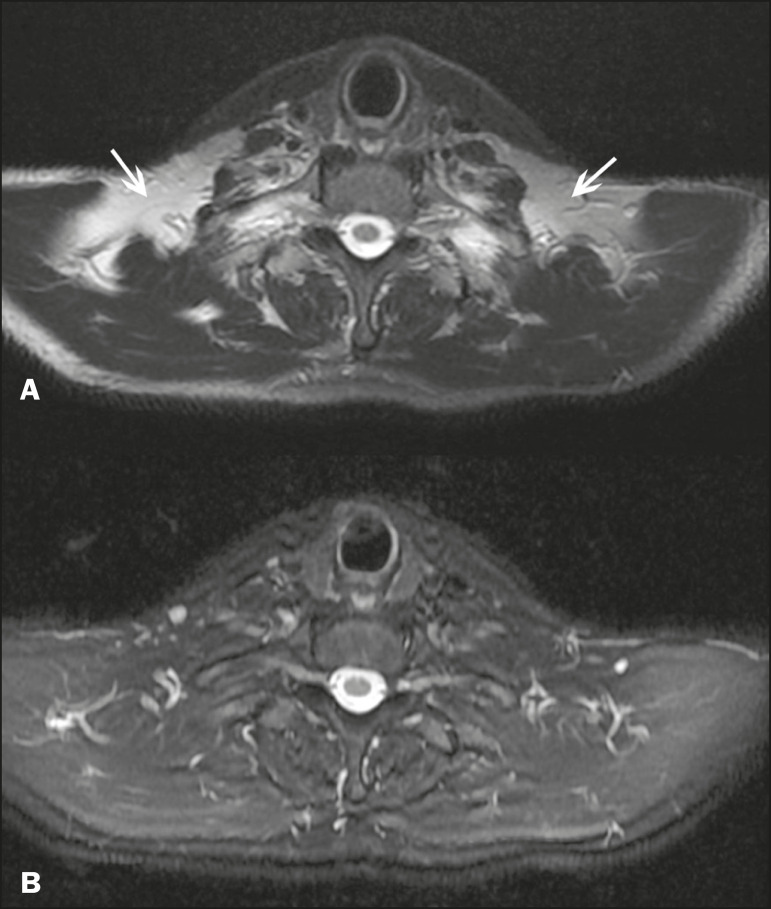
Axial plane images of cervicothoracic transition of a 58-year-old male patient demonstrating insufficient fat suppression in a T2-weighted FSE sequence with fat suppression (A), compared with a T2-weighted IDEAL wateronly sequence (B).
